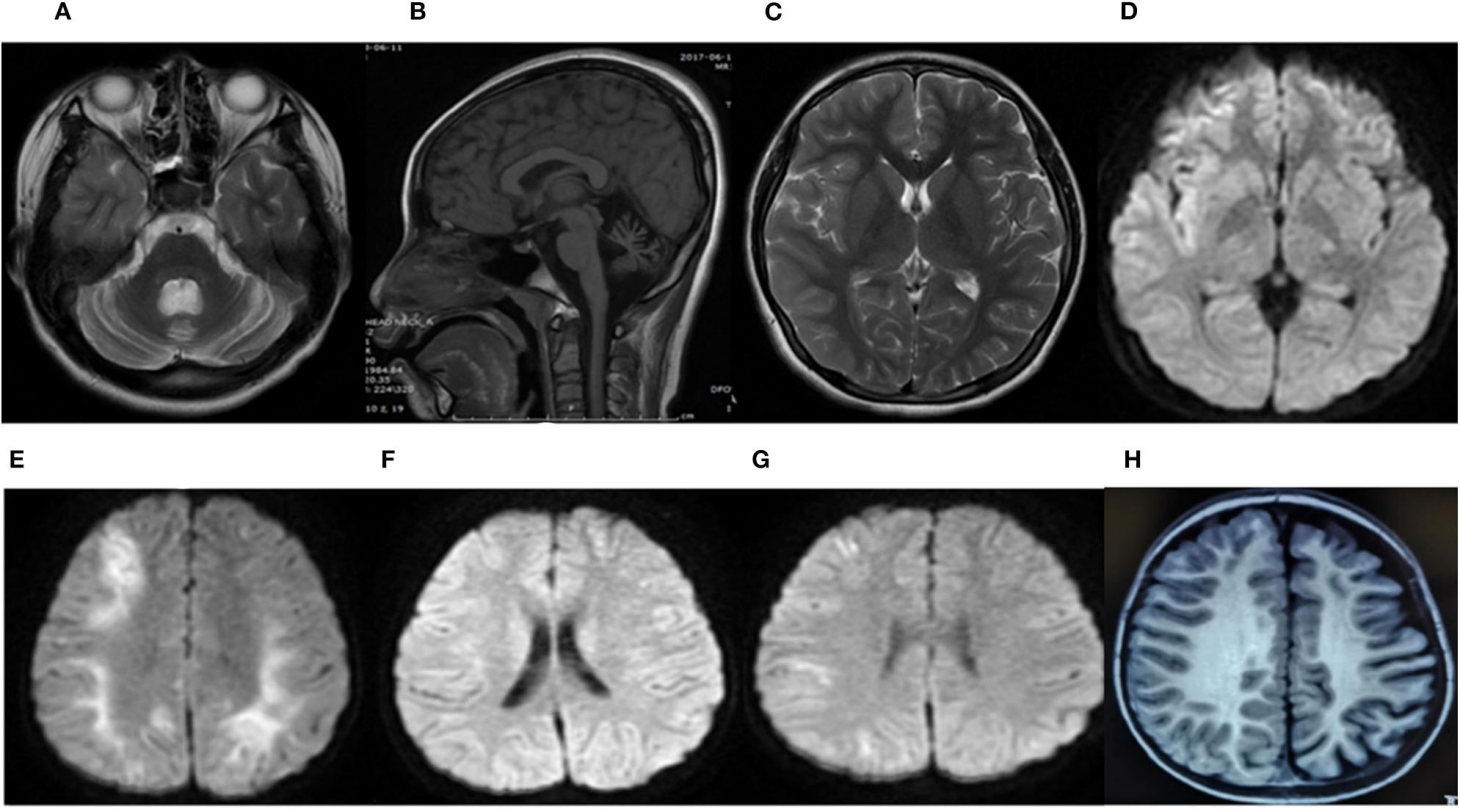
Brain MRI of patient 1 during the last attack at age 13 years (A–D). An axial T2-weighted image (T2W) (A) and a sagittal T1-weighted image (T1W) (B) showed severe cerebellar atrophy. An axial T2-weighted image (T2W) (C) and an axial diffusion-weighted image (DWI) (D) showed a slight hyperintense signal in the frontal, temporal, and occipital region of the right hemisphere. The brain MRI of patient 3 (E–G). The axial diffusion-weighted image (DWI) at age 3 during a severe attack (E), 1 year later (F), and at 6 years old soon after the last episode (G). (E) Revealed restricted diffusion in the bilateral hemisphere. (F) Demonstrated a complete resolution of the cortical lesion. (G) Showed a mild restricted diffusion in the right hemisphere. The brain MRI of patient 6 at 22 months of age (H). An axial T1-weighted image (T1W) showed significant atrophy of the left hemisphere.
